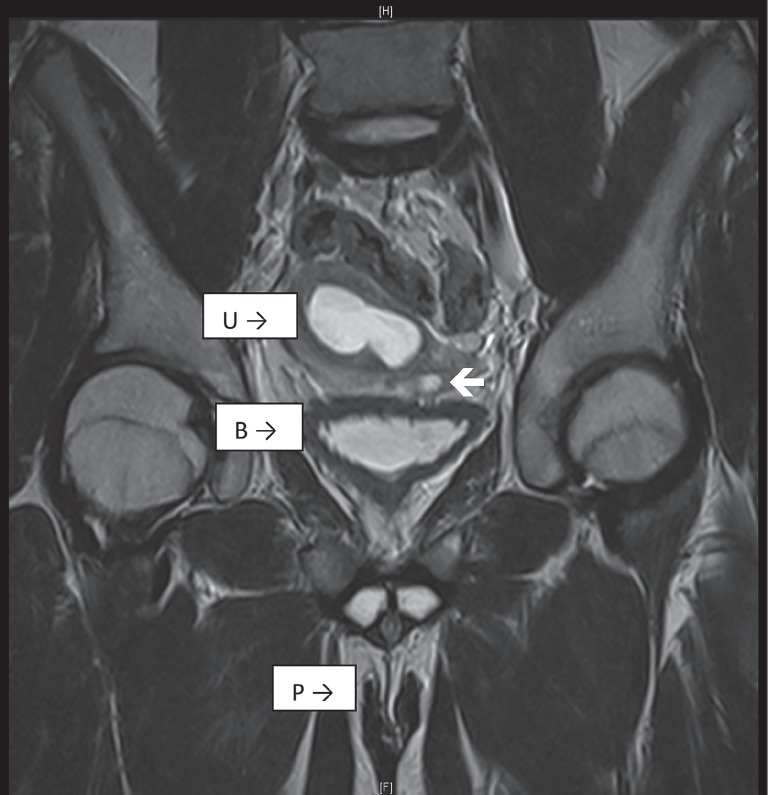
MRI of the pelvis and abdomen of the patient, showing the bladder (B), a single cervix and uterus (U), and two tubular structures at the usual place of corpus cavernosum (P). Structures suggesting the ovaries are not seen. The uterus has two horns, however it has flat fundus which is in favor of incomplete septate uterus. An oval intermediate signal intensity at left hemipelvis, medial to external iliac vessels, is suggestive of a testes. It measured 1.2cm × 1.2cm × 2.3cm.
